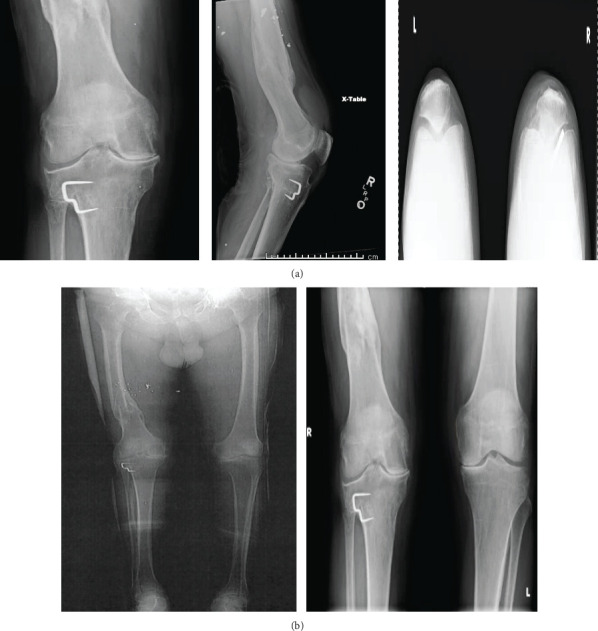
(a) Preoperative anteroposterior, lateral, and sunrise X-ray views displaying tricompartmental osteoarthritis with femoral and tibial deformities, valgus angulation, and tibial osteotomy screws. (b) Preoperative anteroposterior standing bilateral lower extremity X-rays displaying long leg alignment and the mechanical axis with femoral and tibial deformities, valgus angulation, and tibial osteotomy screws.
